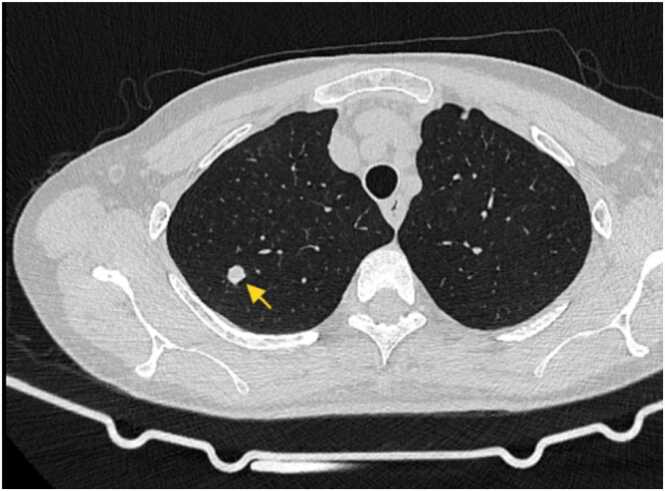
Axial chest CT (lung window): Bilateral secondary pulmonary tuberculosis (arrow), multiple old rib fractures, and aortic wall calcification.
